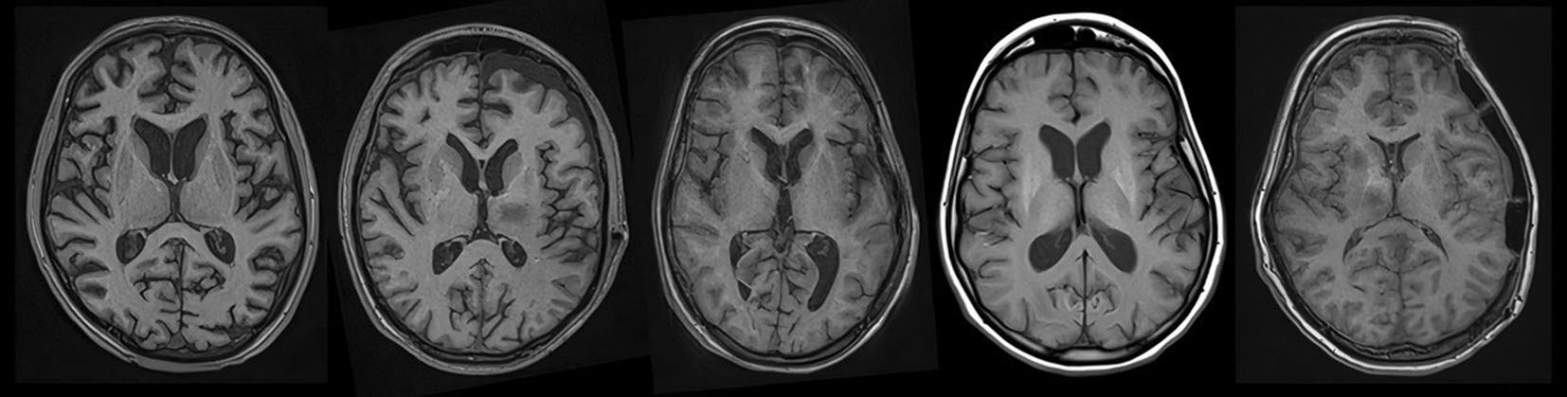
Initial structural MRI scans of included patients showing an absence of any large brain lesion (patients 1–5, from left to right).
